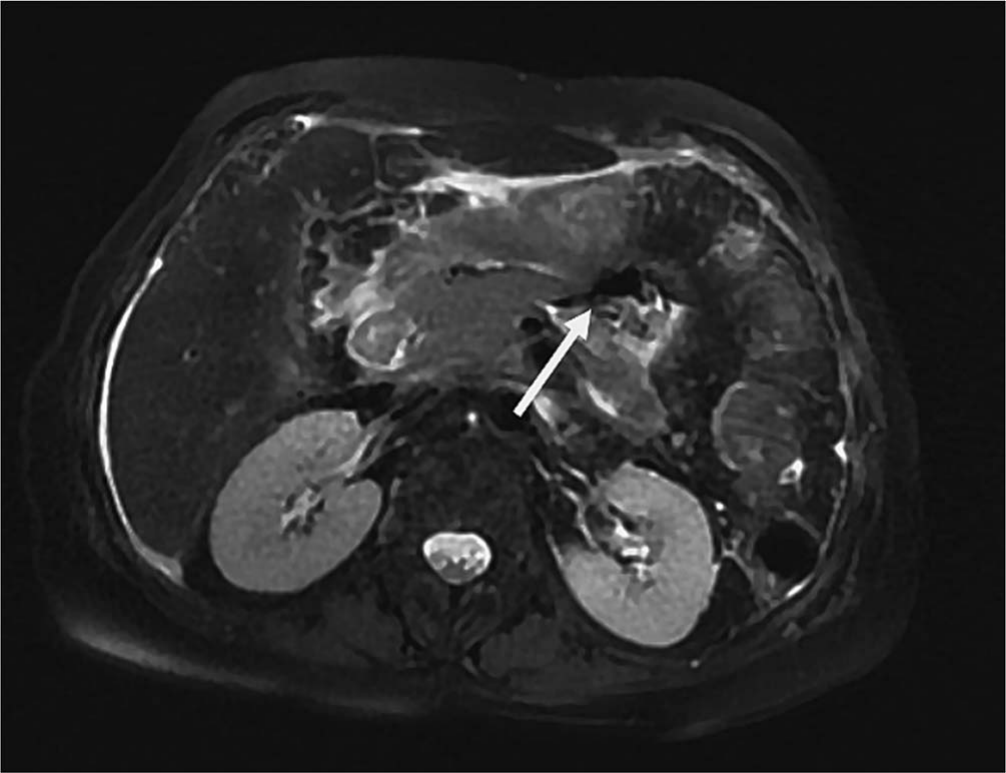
MRI of the abdomen, an axial section, on T2 fat saturated sequence, showing a persistent collection (arrow) in the pancreatic body, as a hyperintense signal and internal T2 hypointense debris, communicating with the main pancreatic duct, measuring 3 cm. Gas bubbles are present in the fluid collection due to a connection with the gastric lumen.Peripancreatic fat stranding is seen.
